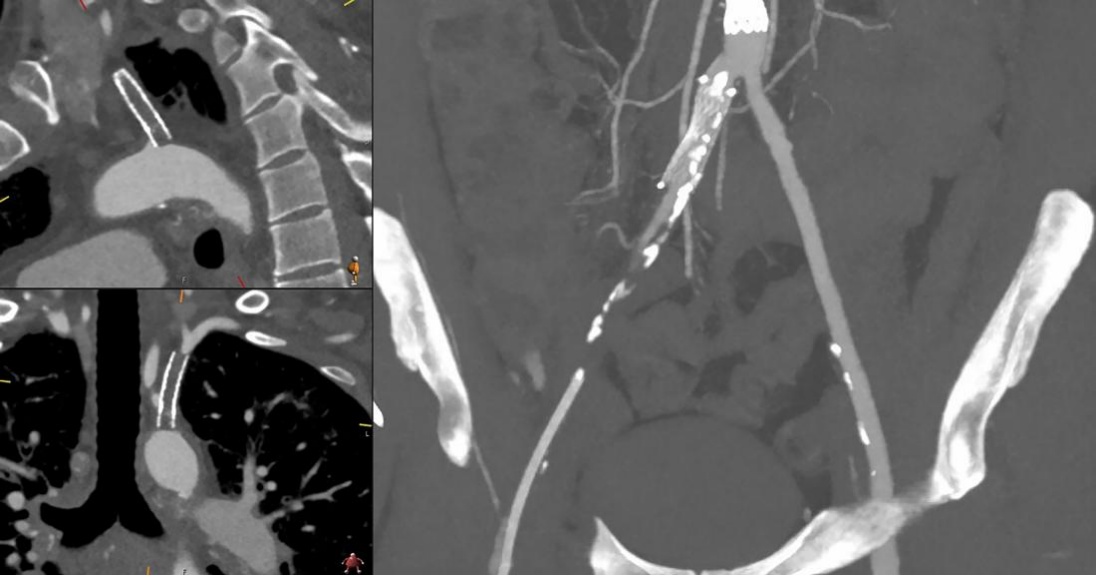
Computed tomography angiography of proximal arteries with peripheral artery disease. Images of the occluded left subclavian artery, both femoral arteries and the left radial artery.
